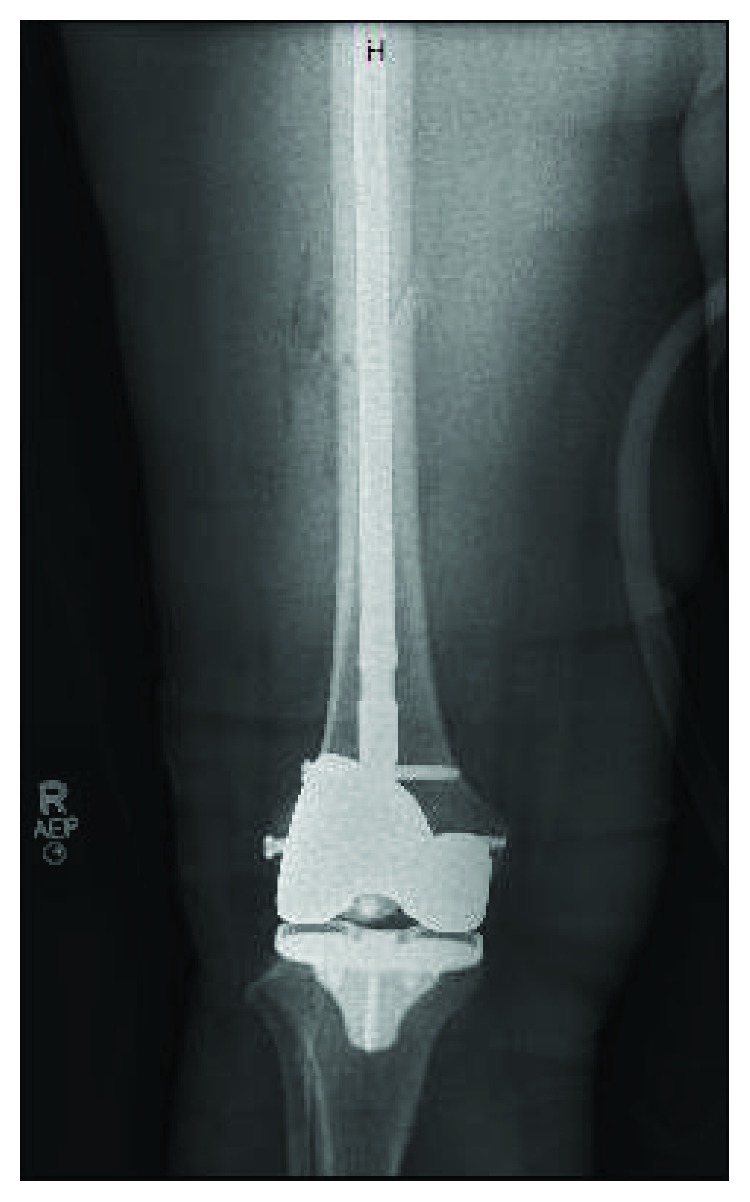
Anteroposterior radiograph of the right femur after intramedullary nailing of the femoral shaft fracture.
